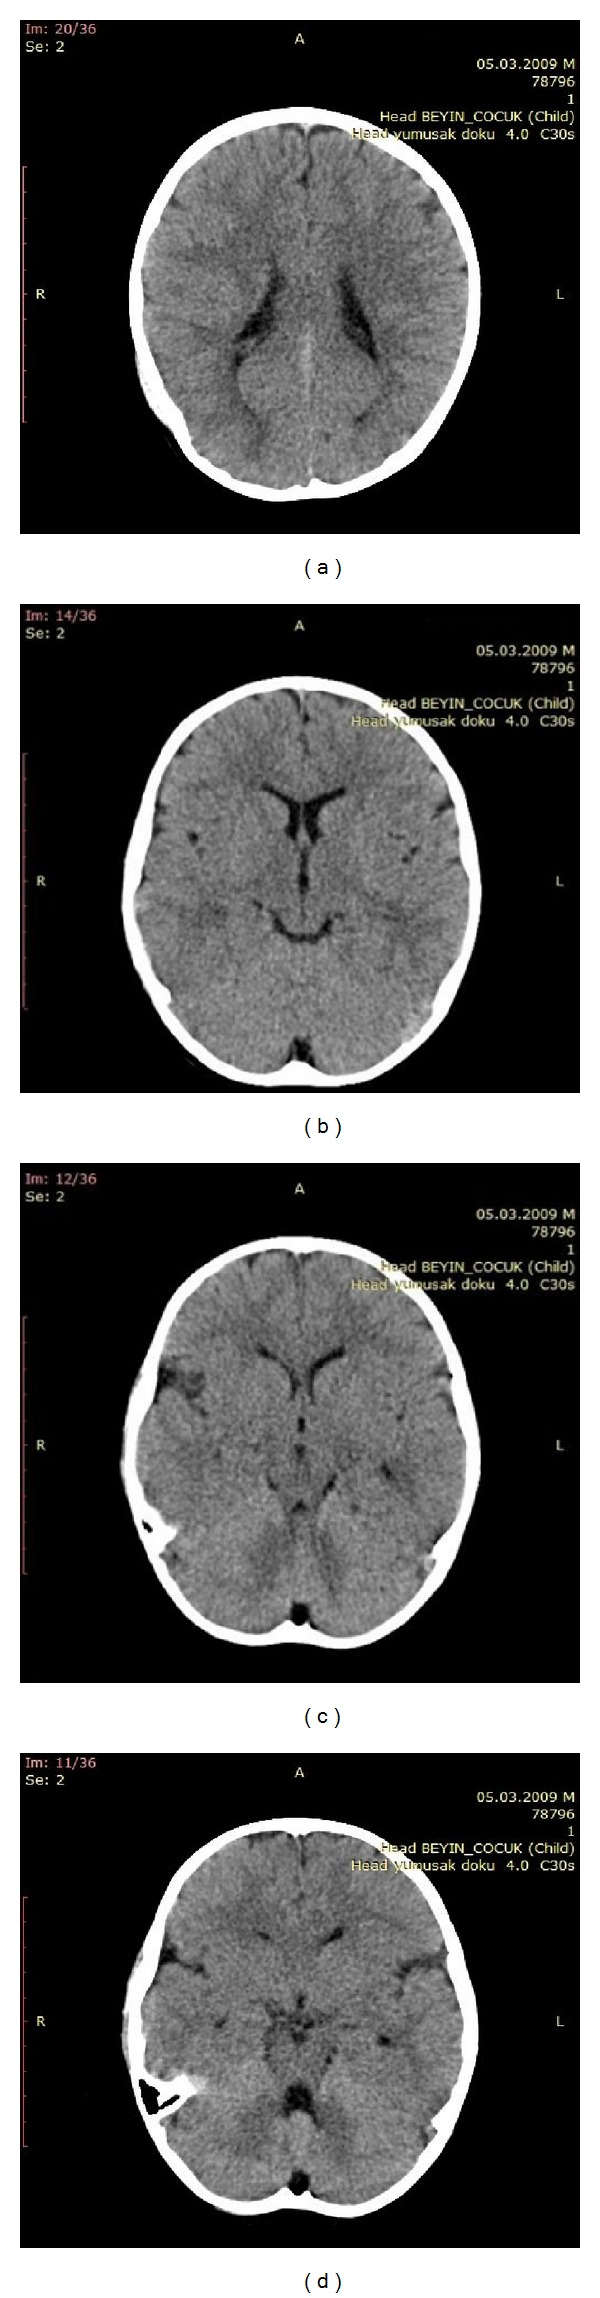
Axial CT image shows complete resolution of the hematoma. (a) Level of mastoid air cells. (b) Level of temporal region. (c) Level of lateral ventricles. (d) Level of centrum semiovale.
